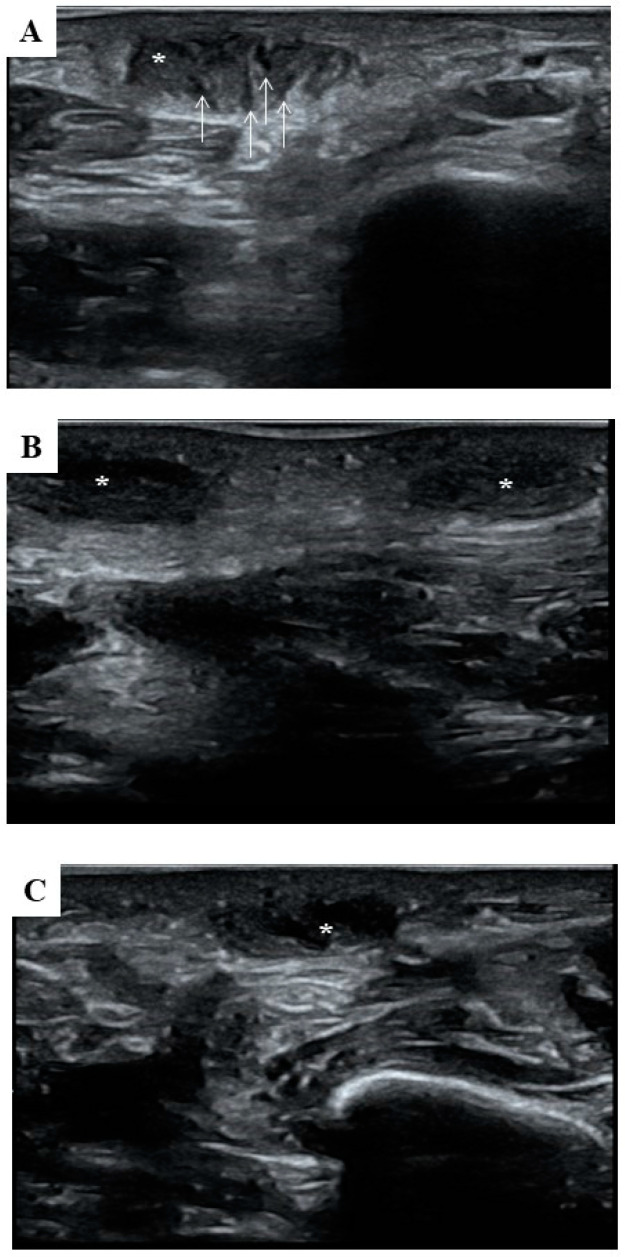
Transverse and longitudinal ultrasonographic images of the plantar nodules. (A) Transverse US imaging of the left foot reveals an oval nodule (asterisk) in contiguity to an intact PA. The nodule shows a mixed echogenicity featured by alternating dark and light bands (arrows). (B) Longitudinal US imaging of the right foot displaying two close hypoechogenic, small fusiform nodules (asterisks) overlying the PA. (C) The transverse US image confirms the hypoechoic and homogeneous structure of the asymptomatic nodule (asterisk) with respect to the surrounding structures and PA.
